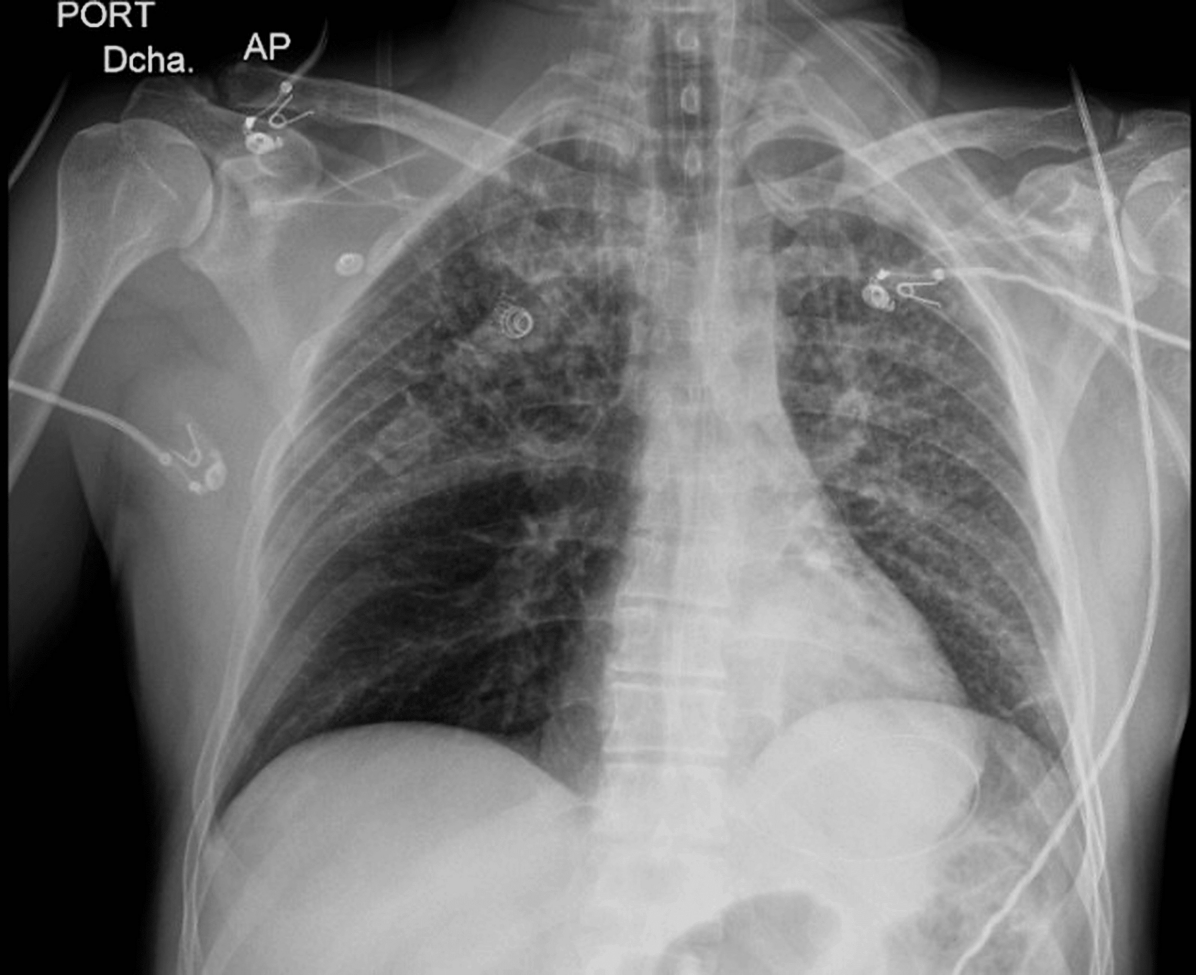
Chest X-ray on ICU day 1 showing mild left-sided alveolar infiltrates
